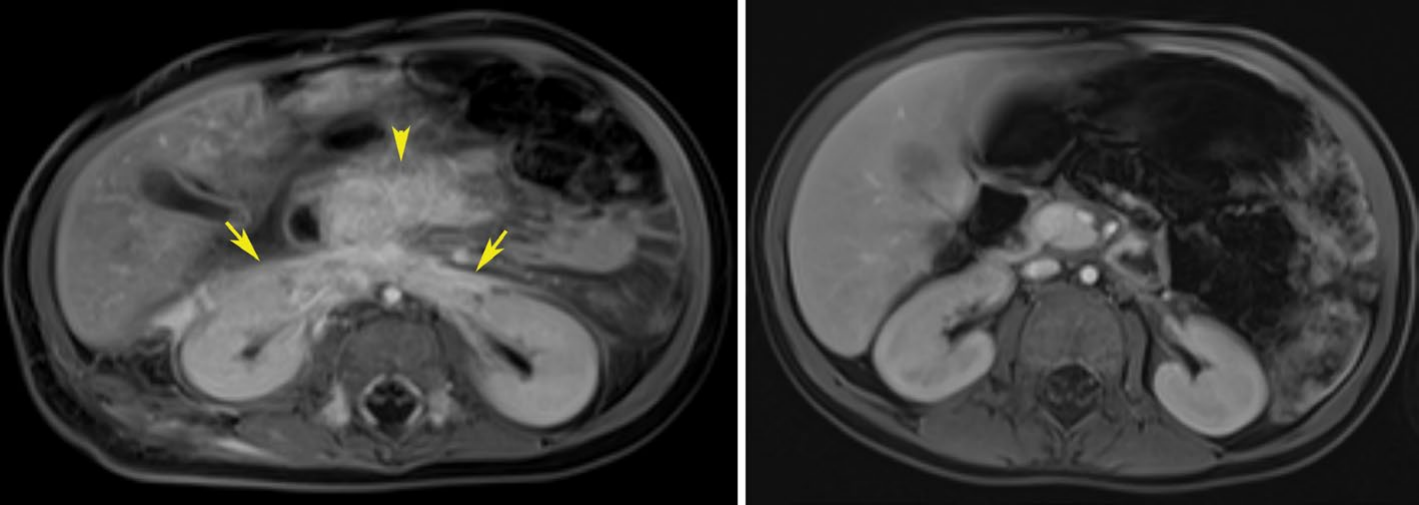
Multiple T1‐weighted axial post contrast images with fat saturation through the upper abdomen. (A) Pre‐therapy MRI at 3 months of age. There is an infiltrative, enhancing mass lesion involving the anterior pararenal space (arrows) with involvement of the pancreatic head and surrounding peripancreatic fat (arrowhead), suspicious for a Kaposiform Hemangioendothelioma (KHE). Not shown is involvement of the mesenteric root and patchy involvement of the right paraspinal musculature. (B) Follow up MRI after 15 months of therapy demonstrates resolution of the infiltrative KHE. The pancreas is now uniform in signal and there is no suspicious enhancement through the mesentery or retroperitoneum.
